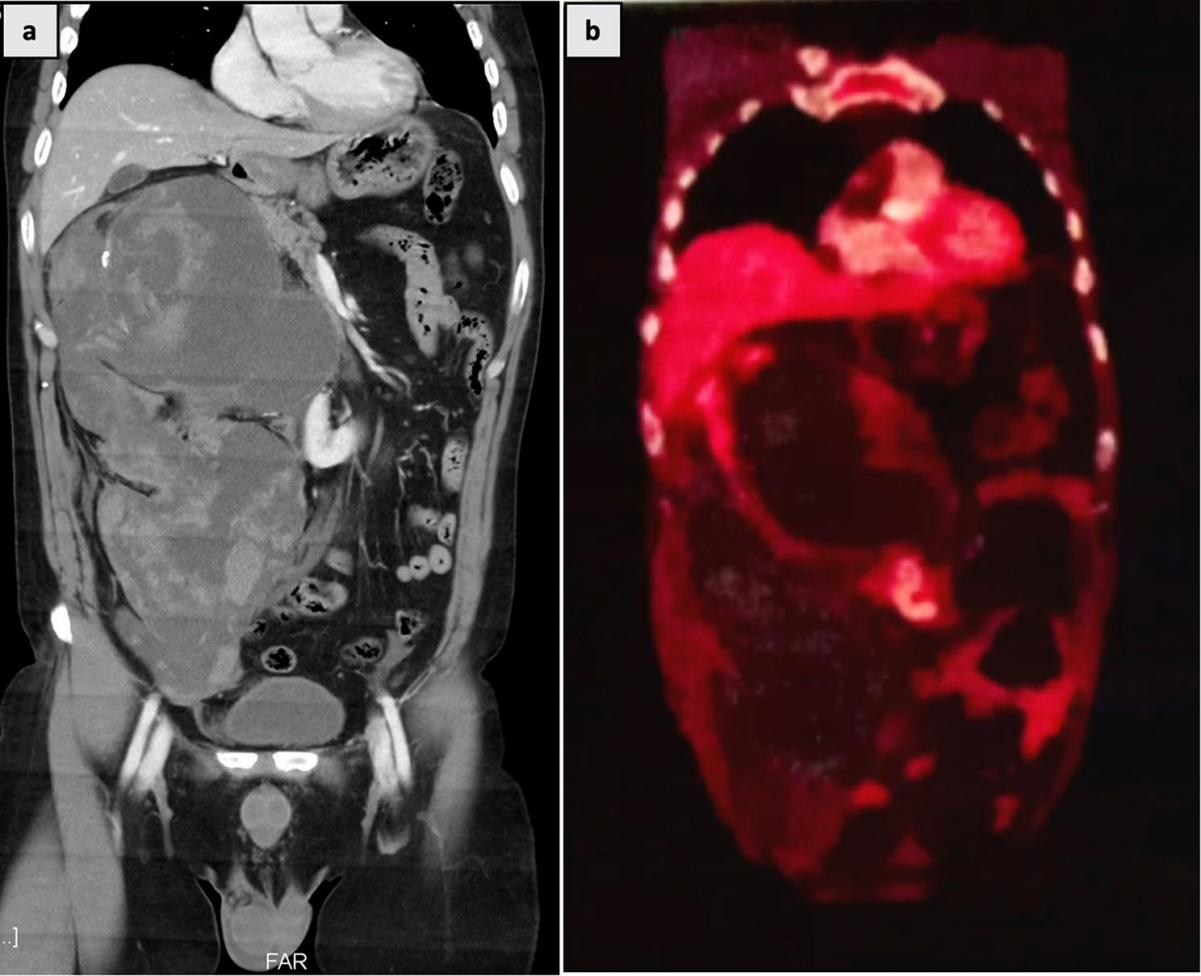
(a) horseshoe kidney morphology with a well-defined multilobulated hypodense non-enhancing cystic lesion in the right hypochondrium extending to the right lumbar region (b) FDG-PET showing an FDG avid soft tissue density thickening noted in the region of the right kidney with a non-FDG avid multiloculated hypodense non-enhancing collections with irregular rim calcifications
